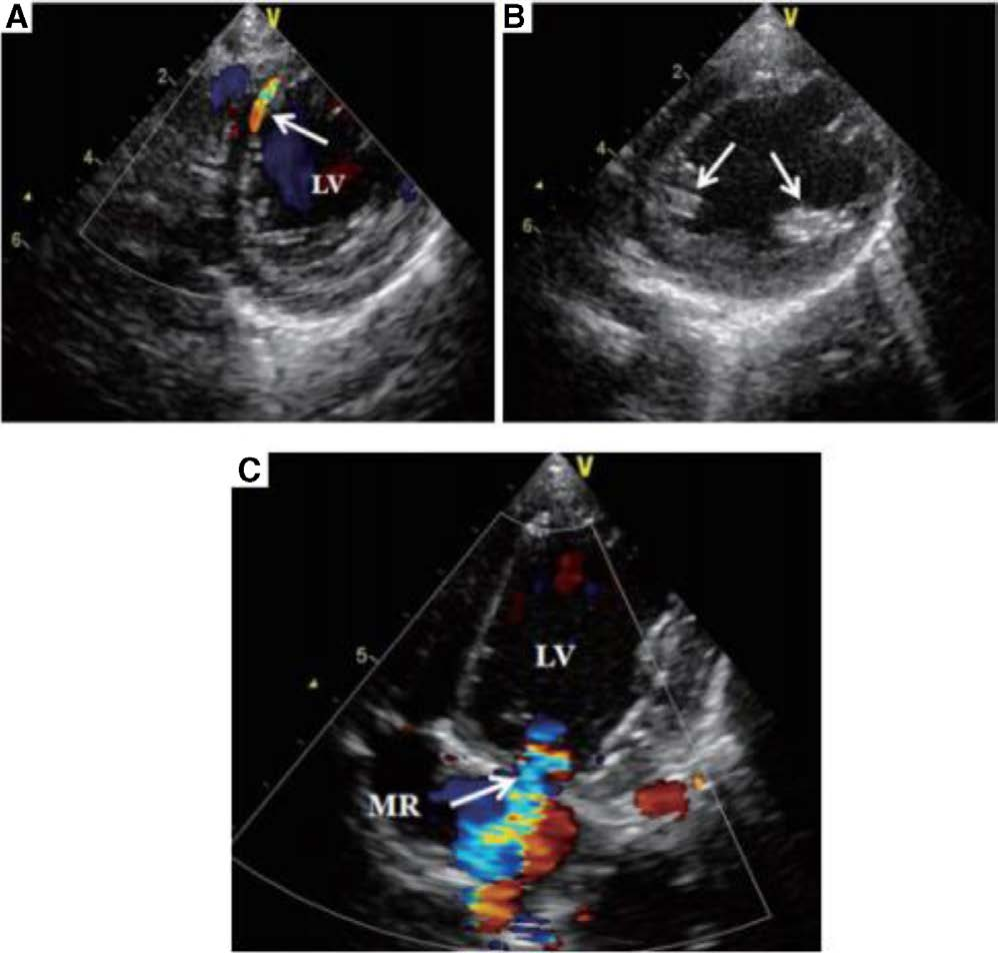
(A) In the parasternal long axis section, color Doppler imaging showed multiple coronary artery collateral branches in the interventricular septum and free ventricular wall (arrow); (B) parasternal short-axis echocardiography showed anechoic enhancement of papillary muscle (arrow); (C) apical 4-chamber echocardiography showed mild mitral regurgitation (arrow).^[7]^
